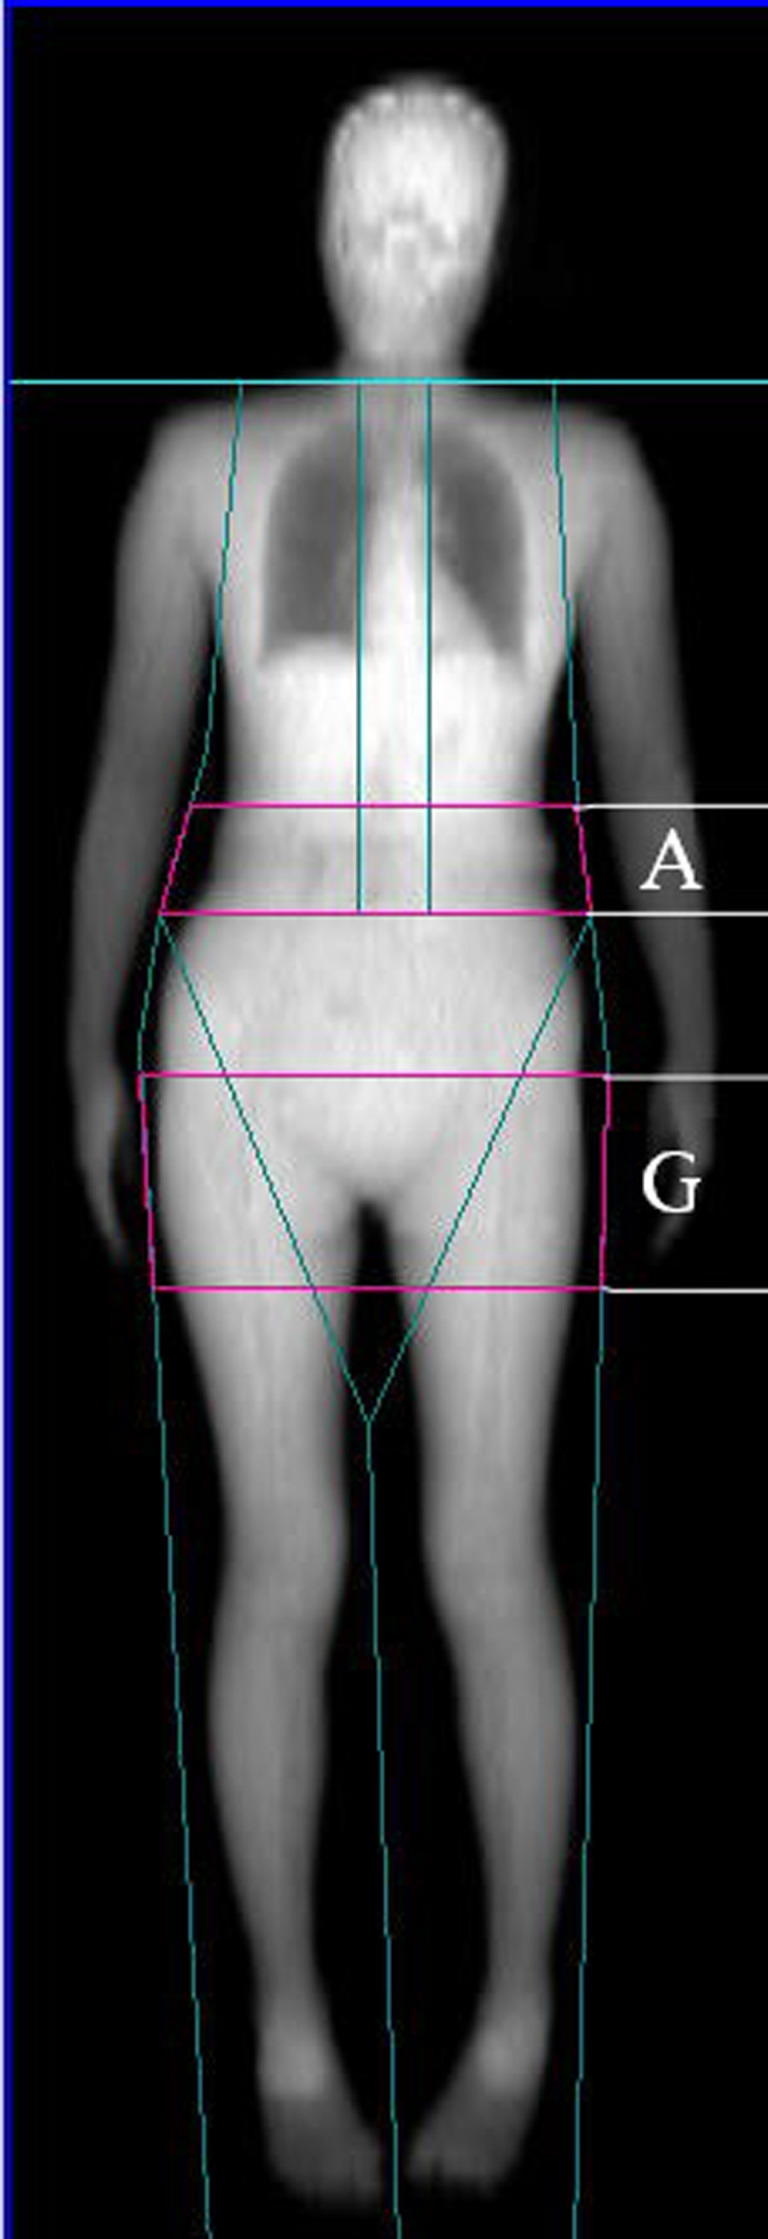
Dual-energy X-ray absorptiometry-based body composition measurement (A: android fat distribution ROI, G: gynoid fat distribution ROI).
